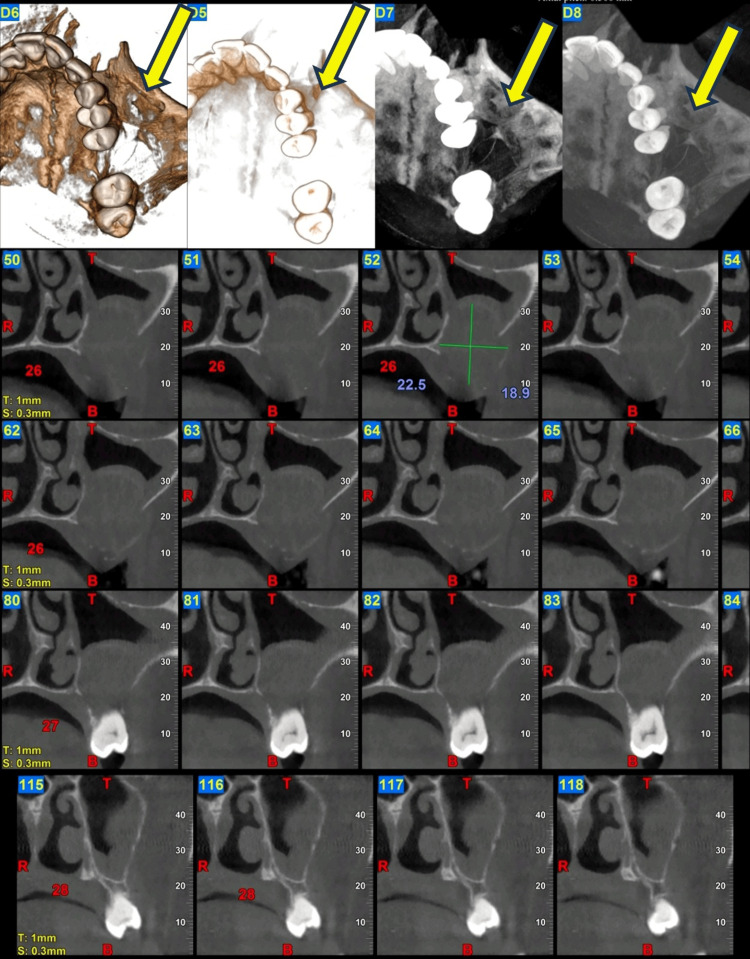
Cone-beam computed tomography (CBCT) reconstruction demonstrating a well-defined mixed radiolucent-radiopaque lesion extending from the region of teeth 23 to 27.
